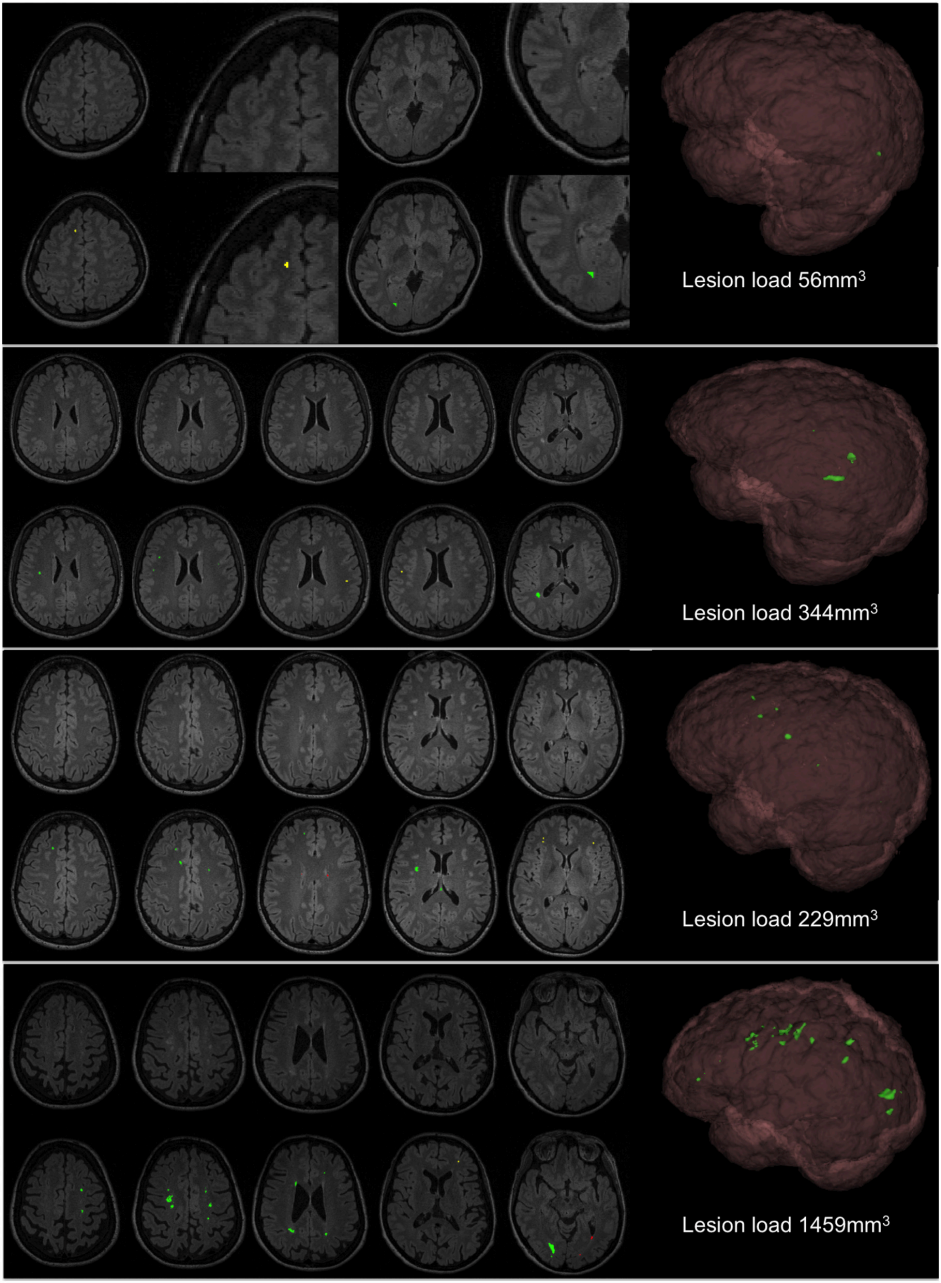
Qualitative results of the approach. First row of each patient shows the original FLAIR image and second row shows the automatic segmentation (green, TP; red, FP; and yellow, FN).
